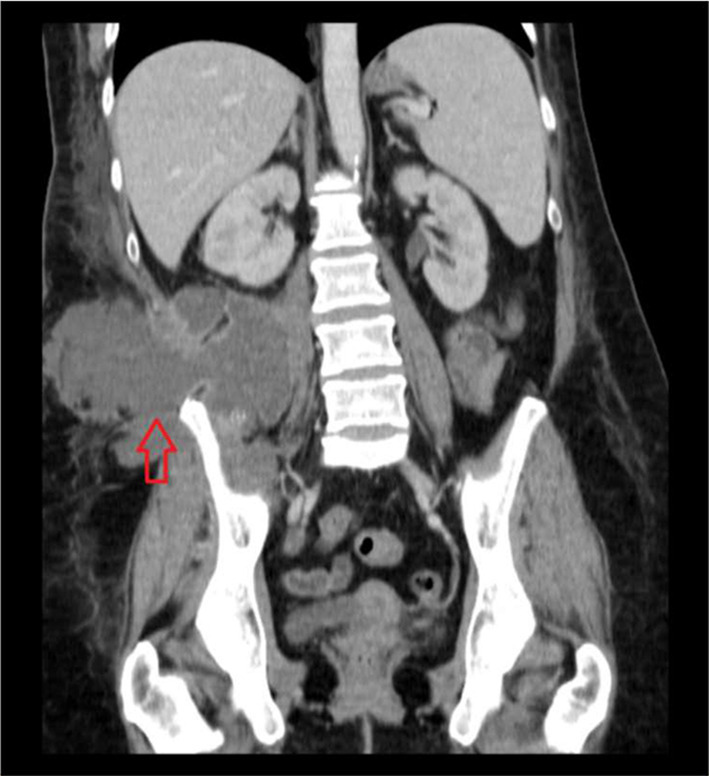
CT abdomen and pelvis (coronal view) shows intra-abdominal abscess extending into the right flank/subcutaneous plane (red arrow).
